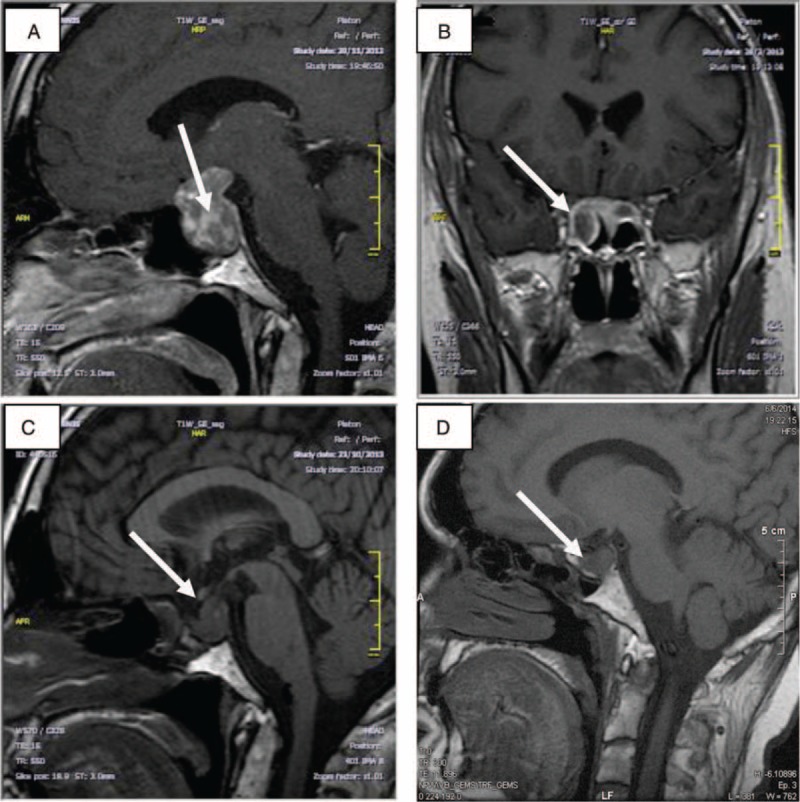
TSHoma pre- and postoperatively and 1 year after long acting octreotide treatment initiation marked by arrows: (A, B) pituitary MRI preoperatively; (C) pituitary MRI postoperatively before long acting octreotide treatment initiation; and (D) Pituitary MRI 1 year after long acting octreotide treatment initiation. MRI = magnetic resonance imaging.
